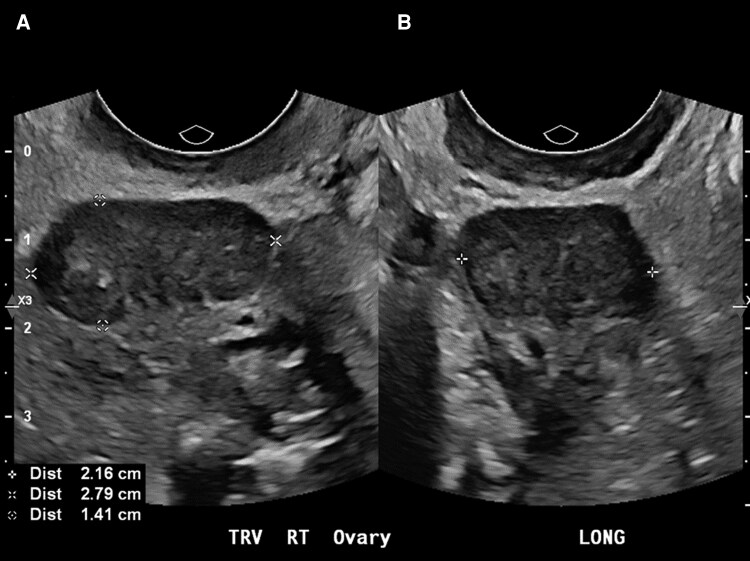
Grayscale transvaginal ultrasound (A, transverse view; B: longitudinal view) demonstrates a hypoechoic mass, measuring 2.2 × 2.8 × 1.4 cm, arising from the right ovary.
